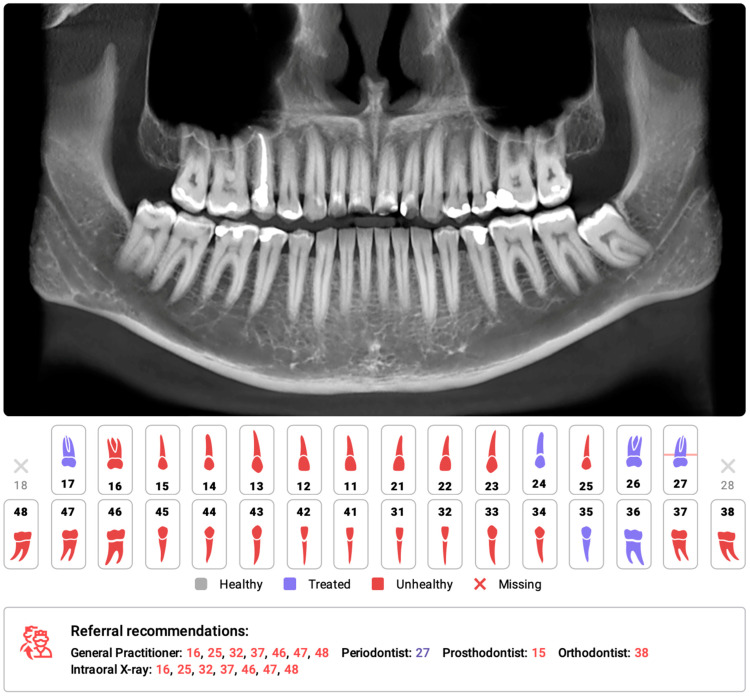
Part of the automatic diagnostic report from a CBCT scan was obtained prior to orthodontic treatment on a 24-year-old male. The software automatically identified the absence of teeth 18 and 28, as well as changes in the remaining teeth, primarily consisting of attrition and the presence of dental fillings. The program has recommended further consultations as necessary.
